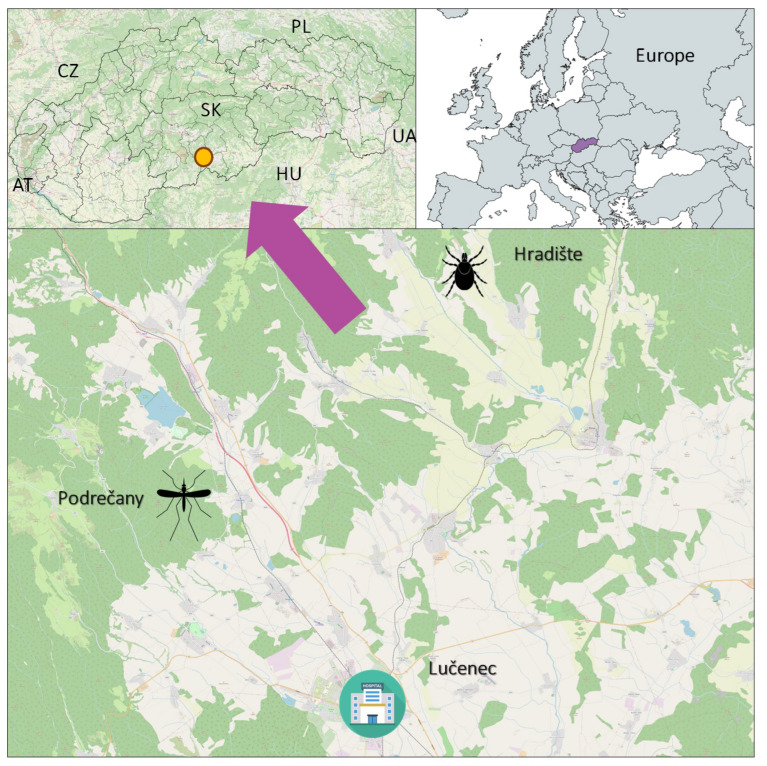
The area of co-circulation of West Nile (WNV), Usutu (USUV), and tick-borne encephalitis (TBEV) viruses in Slovakia, Central Europe. Legend: mosquito icon—WNV, USUV focus, tick icon—TBEV focus, hospital icon—the catchment hospital, orange dot—placement of the area in Slovakia, AT—Austria, CZ—Czechia, HU—Hungary, PL—Poland, SK—Slovakia, UA—Ukraine.
